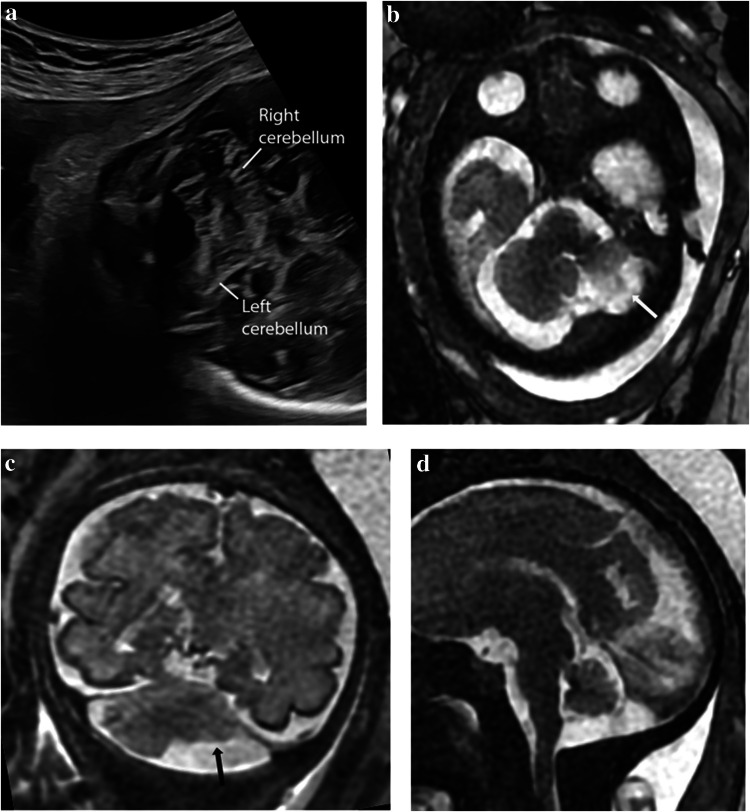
Unilateral cerebellar hypoplasia. Prenatal ultrasound (a) at 31 1/7 weeks shows underdevelopment of the left cerebellar hemisphere with loss of normal architecture. Same-day fetal brain MRI axial (b) and coronal (c) T2 single-shot fast-spin echo (SSFSE) images show a hypoplastic left cerebellar hemisphere (*arrows*). Midline sagittal T2 fast steady-state image (d) demonstrates a normal vermis. Etiology was favored to be an isolated injury. No blood products were appreciable on the heme-sensitive sequence (*not shown*)
